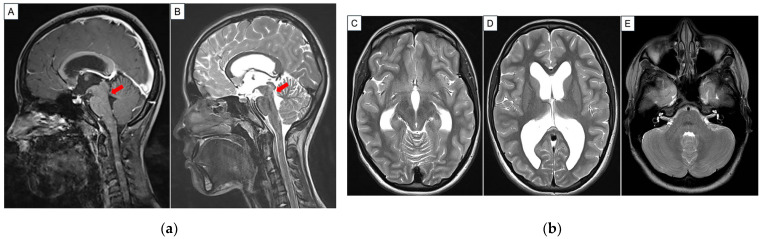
(a) Representative post-contrast T1-weighted [Image (A)] and T2-weighted [Image (B)] sequences of the patient’s MRI brain image in the sagittal direction. The red arrow in both images points to a tissue web within the aqueduct of Sylvius. (b) Representative T2-weighted sequence MRI brain images the in axial direction depicting [Image (C)] slightly enlarged third ventricle and bilateral temporal horns; [Image (D)] bilaterally enlarged frontal and posterior ventricles and [Image (E)] no enlargement of the fourth ventricle. Of note, there is no significant transependymal CSF flow despite the ventricular enlargement.
